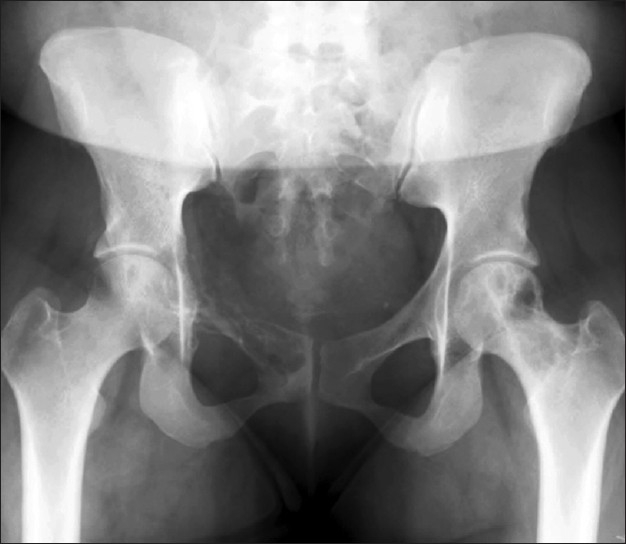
Anterioposterior pelvis radiograph of the right superior pubic ramus and acetabulum osteolytic lesion
